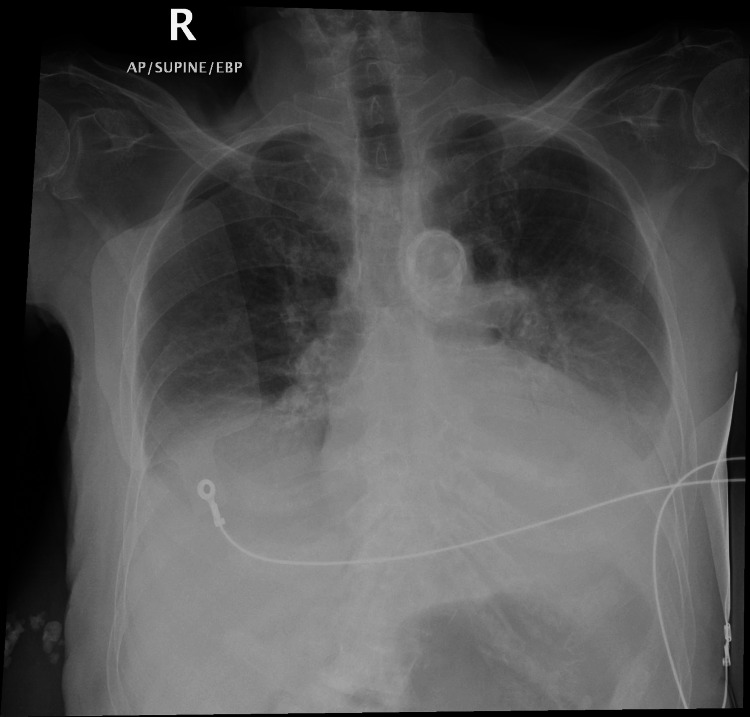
The patient’s chest X-ray indicating pneumonia
